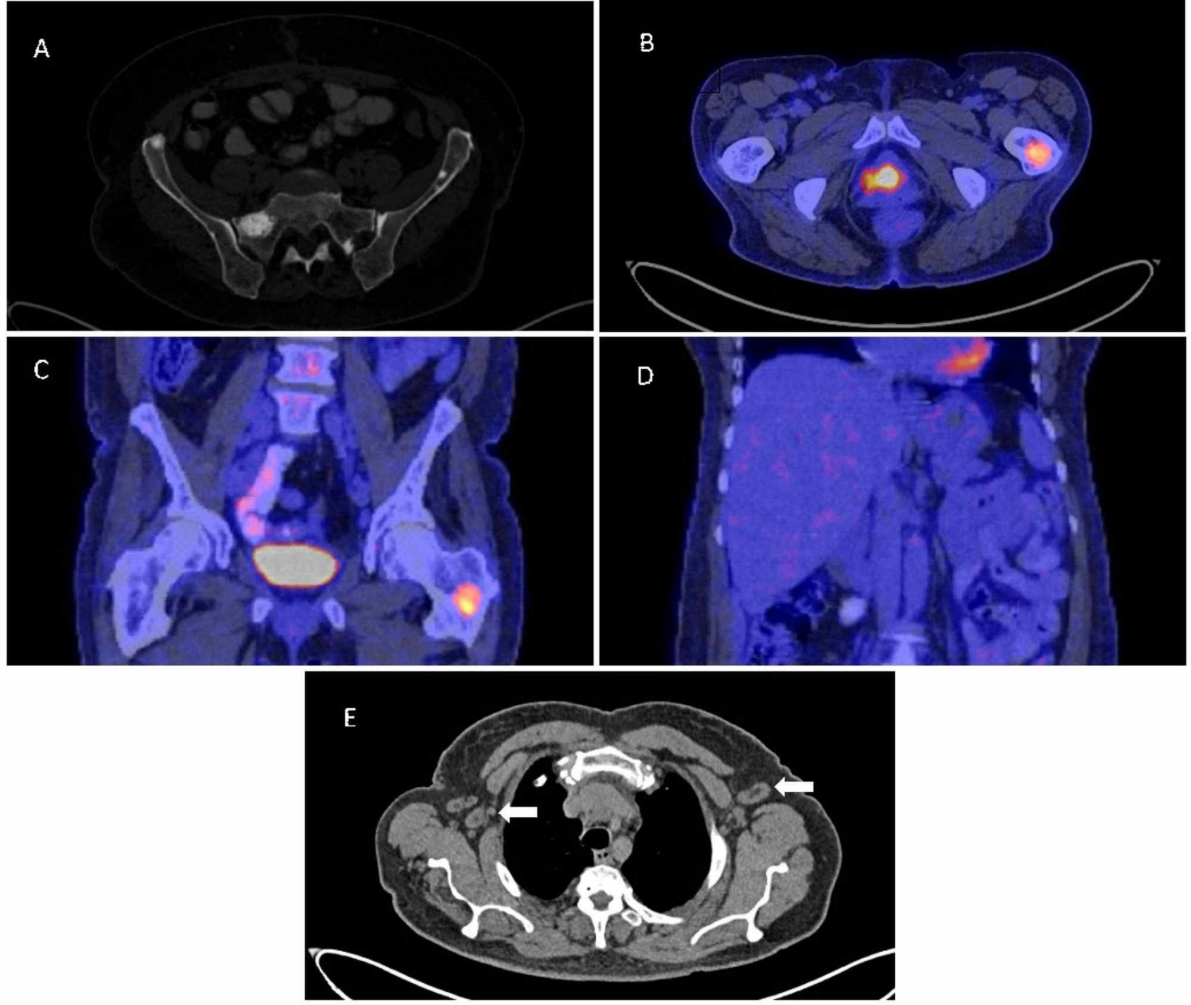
Positron emission tomography CT (PET/CT) findings (A) Sclerotic lesions in the sacrum and iliac bone in cross section. (B) Sclerotic lesion with metabolic activity in the left femur in cross section. (C) Sclerotic lesion with metabolic activity in the left femur in coronal section. (D) Hepatomegaly in coronal section. (E) Bilateral axillary adenomegalies (arrows) in cross section.
